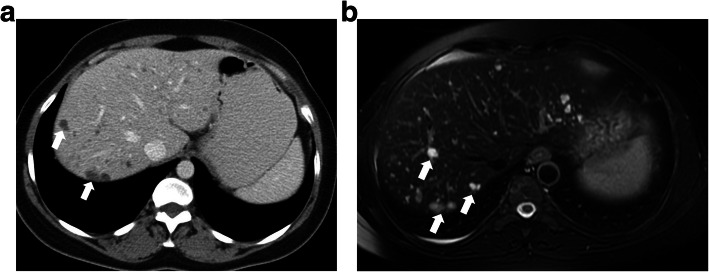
A 48-year-old female patient with known breast cancer underwent abdominal CT scanning for distant metastasis evaluation. a Axial plane post-contrast CT image shows multiple hypodense lesions (arrows) in both liver lobes. b Axial plane fat-saturated T2W MR image shows multiple cystic lesions (arrows) consistent with biliary hamartomas
